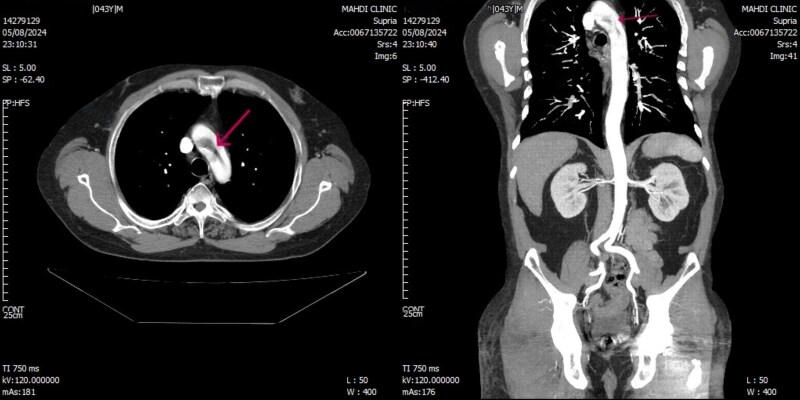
Computed tomography angiography on day 1 demonstrated a large free-floating thrombus within the aortic lumen. The thrombus, measuring 53 mm in craniocaudal length and 13 mm in transverse diameter, appears as a filling defect within the aortic arch and proximal descending aorta (indicated by arrows). It is aligned with the direction of blood flow, with its proximal attachment to the wall of the aortic arch.
